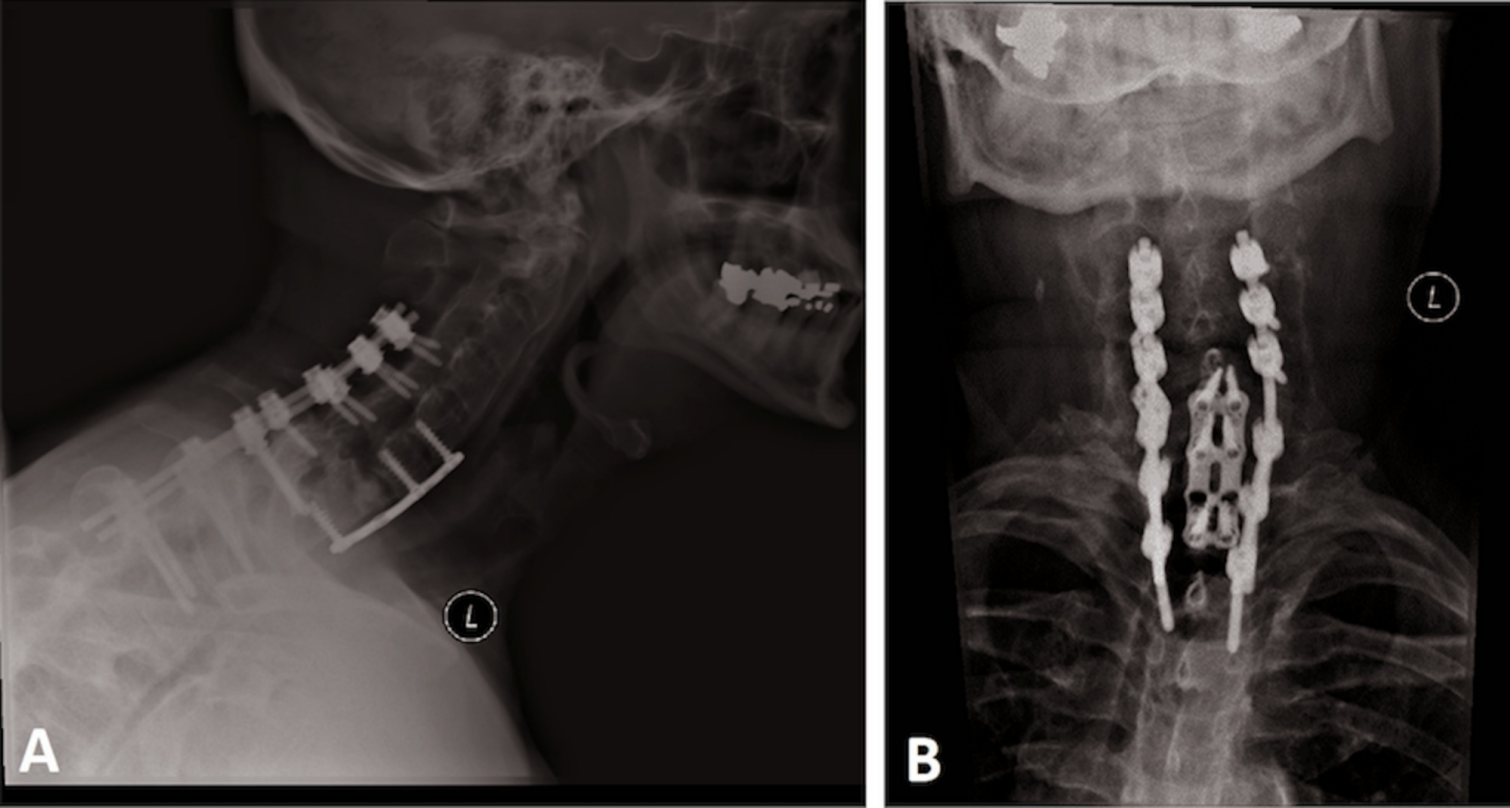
X-rays at most recent follow-up visit Lateral (A) and anteroposterior (B) x-rays of the cervical spine demonstrating healing of the fracture and appropriate hardware placement.
